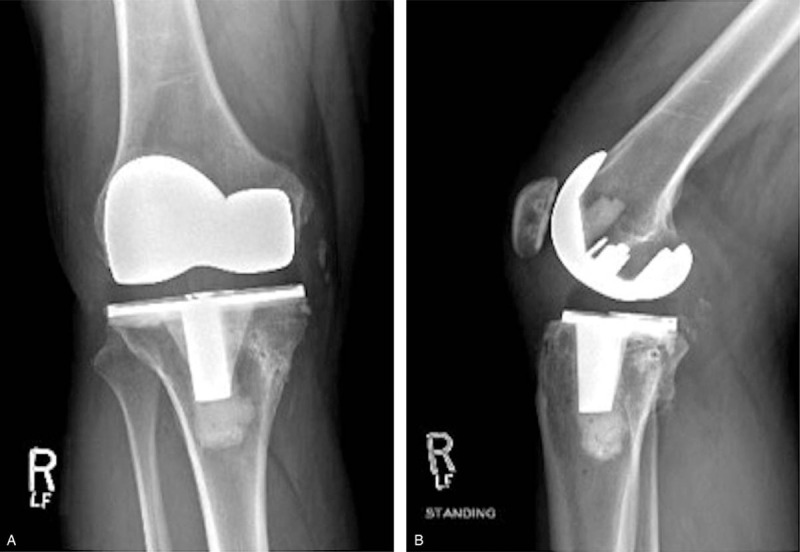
3A: Postoperative AP x-ray demonstrating excellent fixation of the R-TKA. 3B: Lateral x-ray of Figure 3A.
